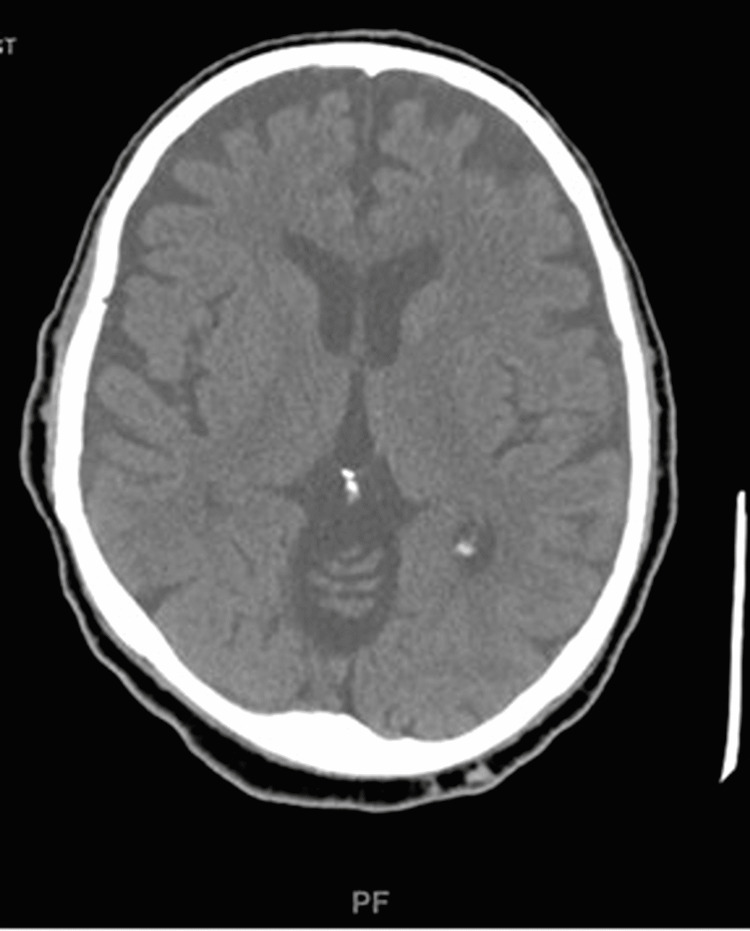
CT Brain without contrast. CT of the brain was performed without intravenous (IV) contrast showing small bilateral frontal crescentic shaped mixed density subdural hematomas measuring up to 7 mm on the left and 5 mm on the right in maximal thickness and no evidence of ischemic infarct.
